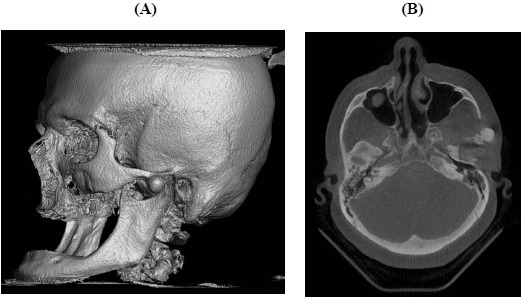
Cone Beam Computed Tomography scan. (A) Axial image showing a round well-circumscribed radiopaque lesion originating from the lateral surface of the left zygomatic arch. (B) Three-dimensional reconstruction image visualizing a well-circumscribed radiopaque structure located on the lateral border of the left zygomatic arch.
